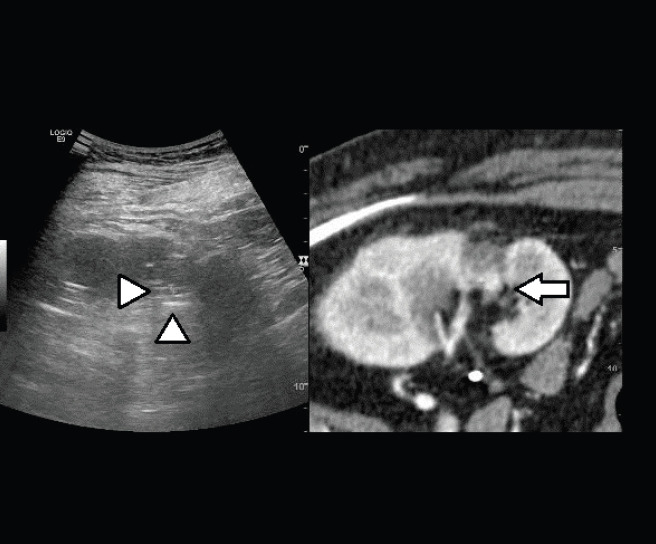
Fusion imaging combining real time US with CT images: CT scan shows the hypervascular nodule deeply in the scar of the previous treatment (arrow); the lesion is not clearly visible at US (heads of arrow).
